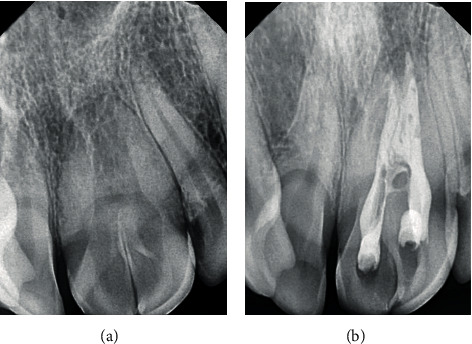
(a) Initial radiograph of tooth #21 before the endodontic intervention. (b) Radiograph of tooth #21 after the endodontic treatment, with the set of the 3 mm apical plugs of MTA.
